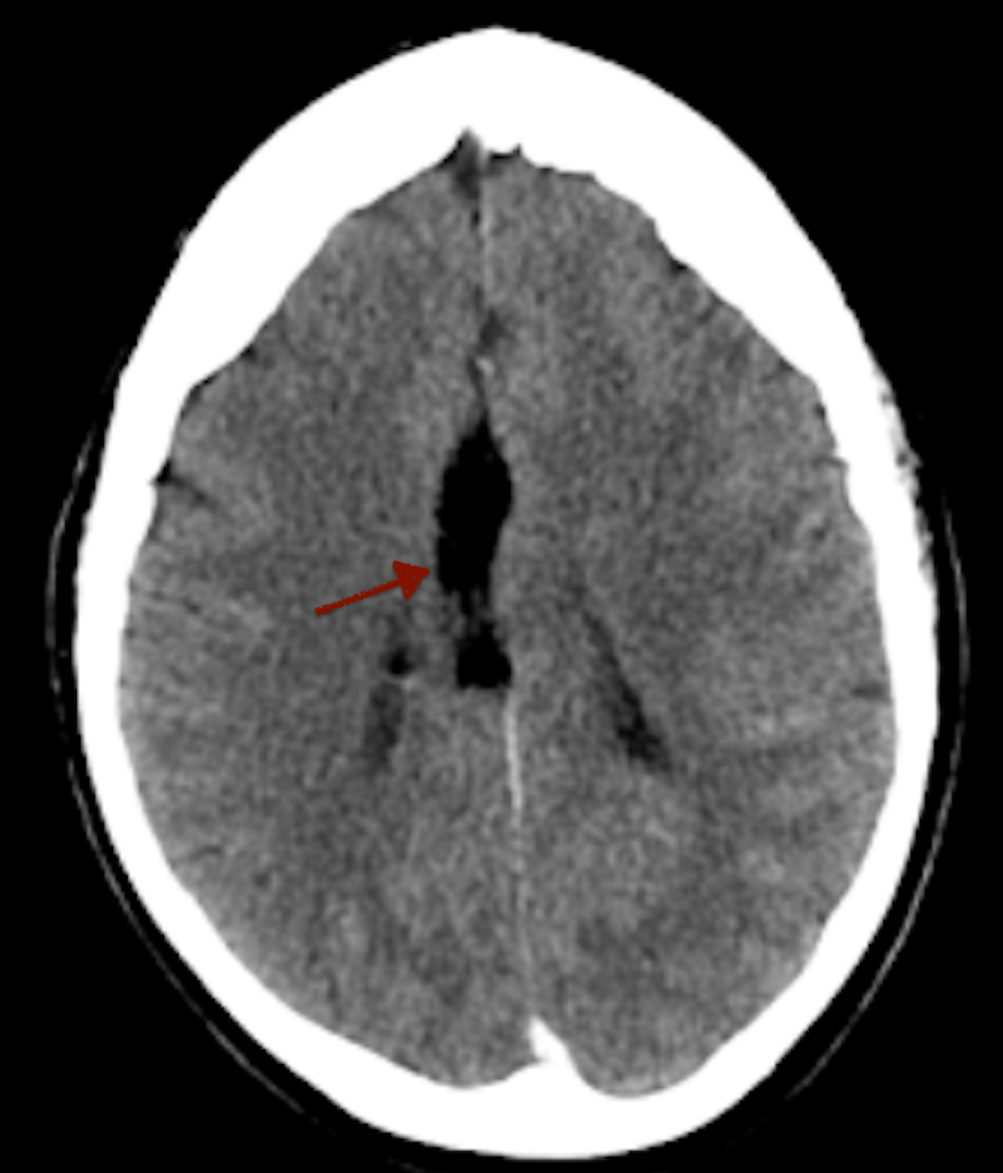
Axial non-contrast CT of the head Axial non-contrast CT of the head demonstrates a midline hypodense fat-attenuating lesion (red arrow) measuring up to 1.5 cm in axial diameter.
